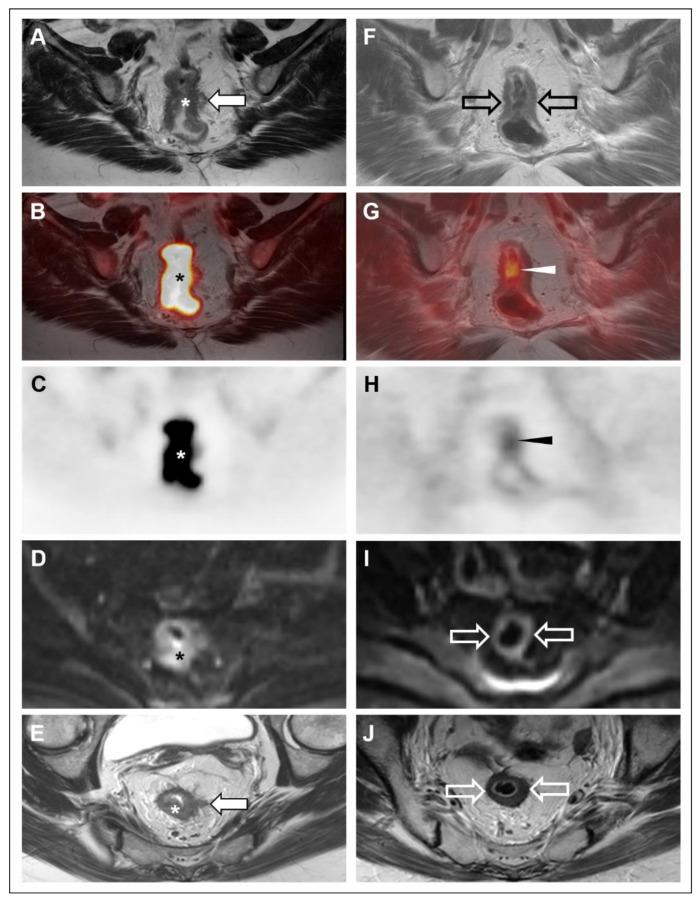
53-year-old woman with rectal adenocarcinoma (Subject #3) underwent FDG-PET/MRI at initial staging (A–E) and following TNT (F–J). Oblique coronal high-resolution coronal T2-weighted images (A,F); oblique coronal high-resolution coronal T2-weighted images with PET fusion (B,G), oblique coronal attenuation-corrected PET images (C,H), axial diffusion-weighted images (b = 1000 s/mm^2^) (D,I), oblique axial high-resolution T2-weighted images (E,J) are shown. At initial staging, a hypermetabolic, diffusion-restricting mid-rectal mass (*) with tumor extension into the mesorectal fat (closed arrows) was noted, along with several suspicious mesorectal nodes (not shown). Following TNT, there was no definite residual disease on MRI (open arrows). Both readers assigned an mrTRG of 1–2. However, PET showed residual focal hypermetabolism (arrowheads) in the treated area. Both readers assigned a pmrTRG of 2 based on possible residual tumor on PET. Subsequent lower endoscopy revealed a stricture of the treated segment that could not be traversed, though biopsies of the accessible portions were benign. Based on the suspicion of residual disease on the PET, the patient underwent a low anterior resection, with surgical pathology revealing residual disease (ypT2 ypN0).
